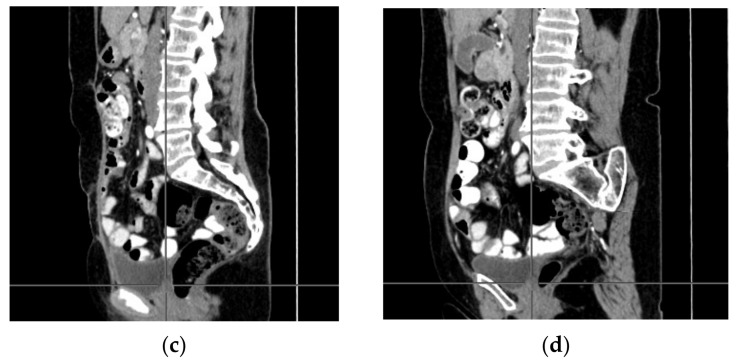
(a–d) CT scans performed at the beginning of olaparib treatment.
